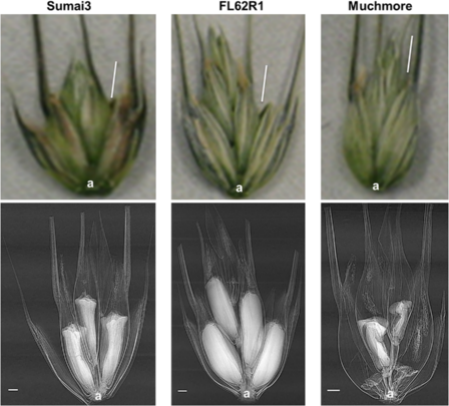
Images of control and healthy florets in the spikelets of wheat cultivars using phase contrast X-ray imaging at 10 days after inoculation with FHB. The florets were mounted on a kapton tape and X-ray images were recorded at 18 keV using a 8.75 μm resolution detector. Red inclined bar: the limit of the first fertile floret; (a): the rachilla. Scale bars indicate 1 mm.
